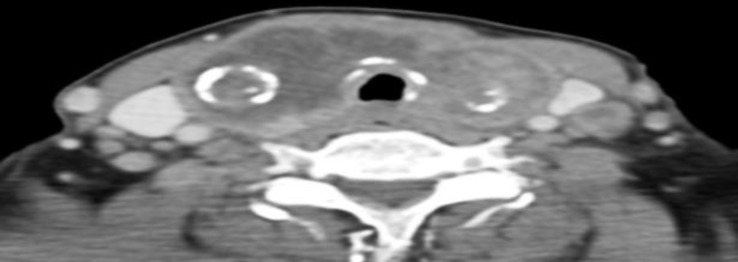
CT showing hypodense mass occupying right thyroid gland, across the isthmus to the left thyroid lobe with a ring calcification feature on right lobe
